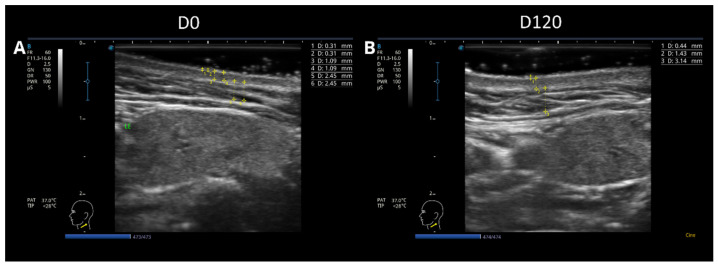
US evaluation of cutaneous and subcutaneous layers before and after treatment with the STIIM UP Protocol. High-resolution ultrasonographic images of the treated region acquired at baseline (D0; (A)) and 120 days post-intervention (D120; (B)). Linear calipers mark the distinct boundaries of the tissue layers, demonstrating a comprehensive structural reorganization. The yellow “+” symbols indicate the endpoints of the ultrasound calipers used to measure tissue thickness, and the numerical values are reported in millimeters (mm). In panel A, measurements 1–2 correspond to the epidermis, 3–4 to the dermis, and 5–6 to the subcutaneous tissue; in panel B, measurements 1, 2, and 3 correspond to the epidermis, dermis, and subcutaneous tissue, respectively. The alphanumeric information displayed on the left side of each image represents the ultrasound system acquisition and display settings and does not correspond to study measurements or outcomes. Quantitative analysis reveals a substantial increase across all structural layers, with measurements recorded in millimeters (mm): epidermal thickness increased from 0.31 mm at D0 to 0.44 mm at D120; dermal thickness increased from 1.09 mm at D0 to 1.43 mm at D120, reflecting neocollagenesis and extracellular matrix remodeling; and subcutaneous tissue thickness increased from 2.45 mm at D0 to 3.14 mm at D120, demonstrating an overall optimization of the skin bed architecture.
